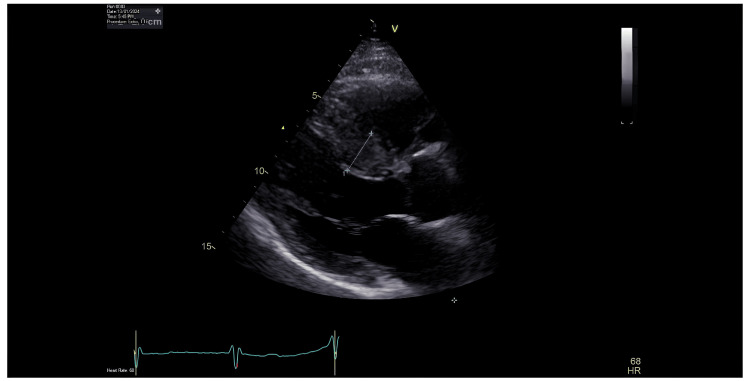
Echocardiogram - parasternal long-axis view Pronounced asymmetric septal hypertrophy.
